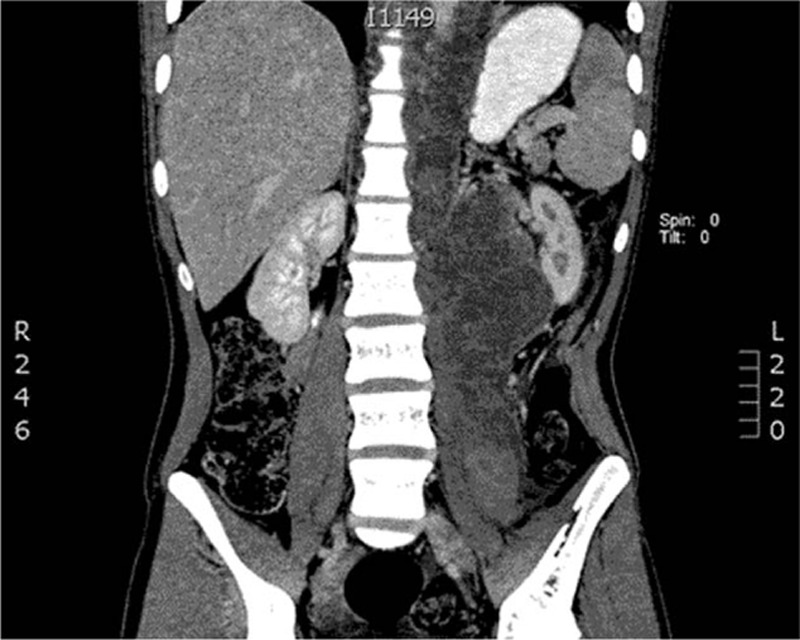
Computed tomography—massive metastases in retroperitoneal space and posterior mediastinum.
